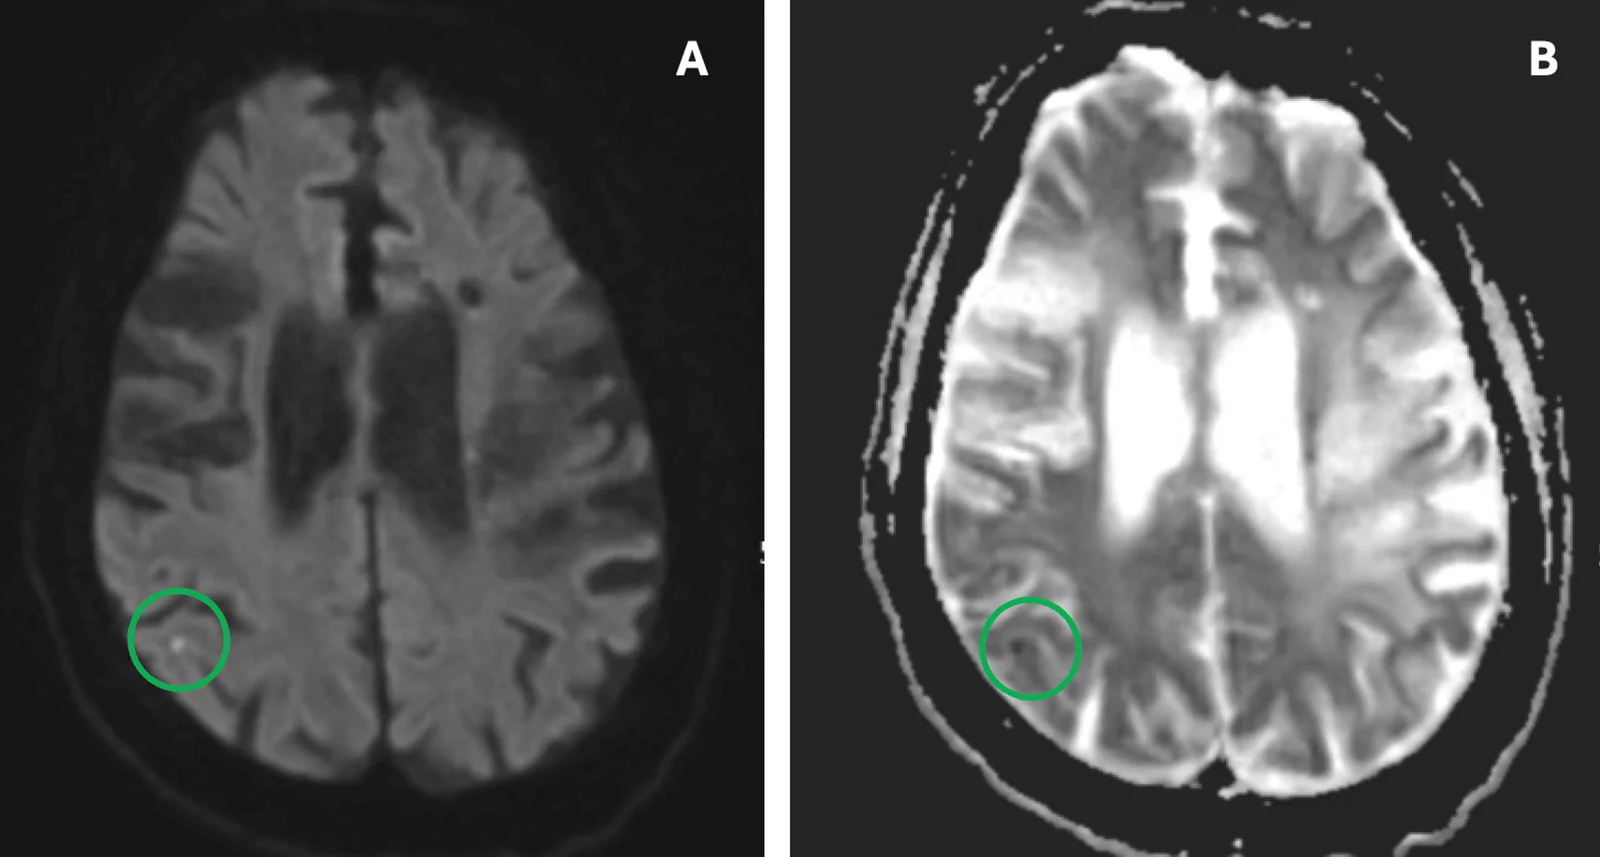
MRI brain with and without contrast demonstrating acute punctate infarct A) Diffusion-weighted imaging demonstrating a punctate area of restricted diffusion in the right parietal cortex (green circle). (B) The corresponding apparent diffusion coefficient (ADC) map shows low ADC signal in the same region (green circle), confirming a new acute ischemic stroke.
